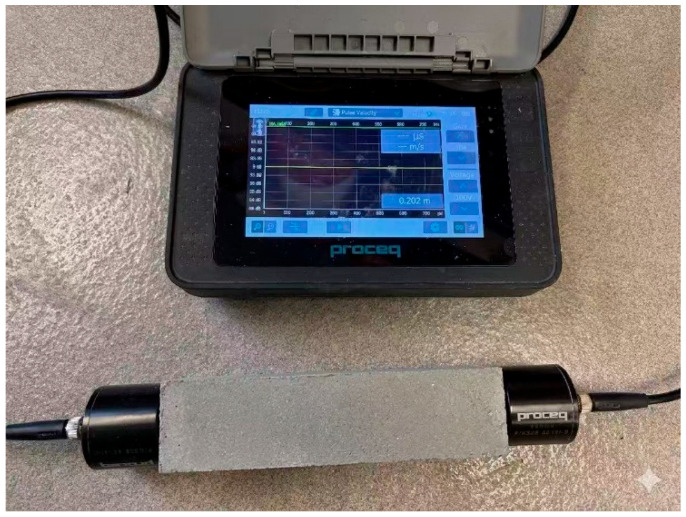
The ultrasonic pulse velocity equipment for dynamic modulus.
